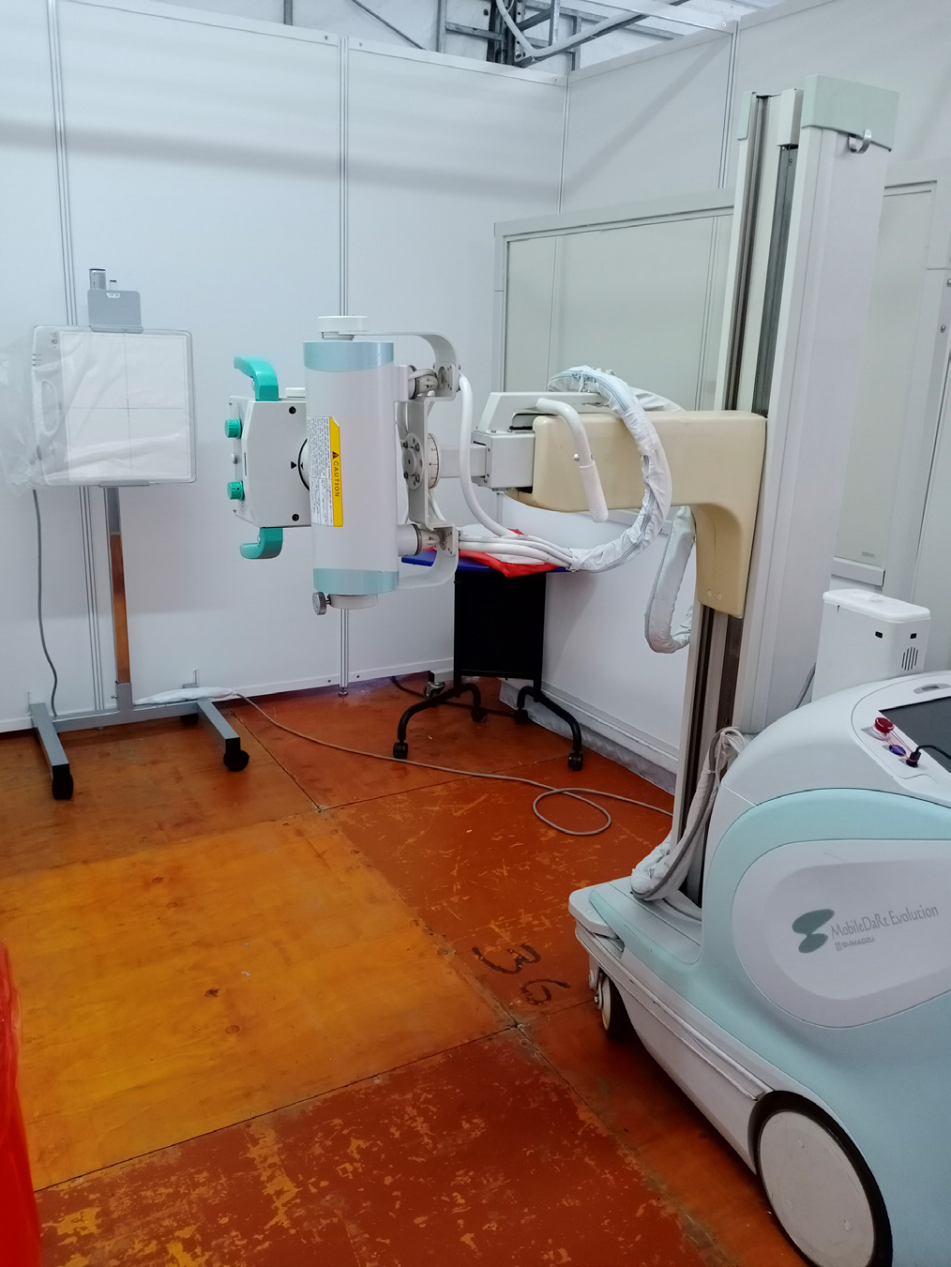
Photograph of the temporary X-ray cubicle within the ESW shows a portable DR X-ray machine and adjacent lead shield. The X-ray detector is covered with disposable plastic and cleaned after every patient
